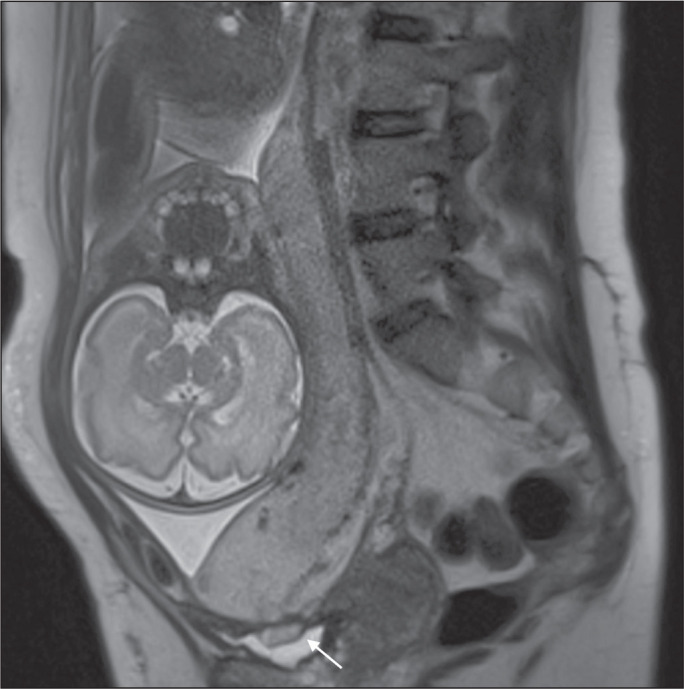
A 30-year-old patient with total placenta previa. Sagittal T2-weighted
